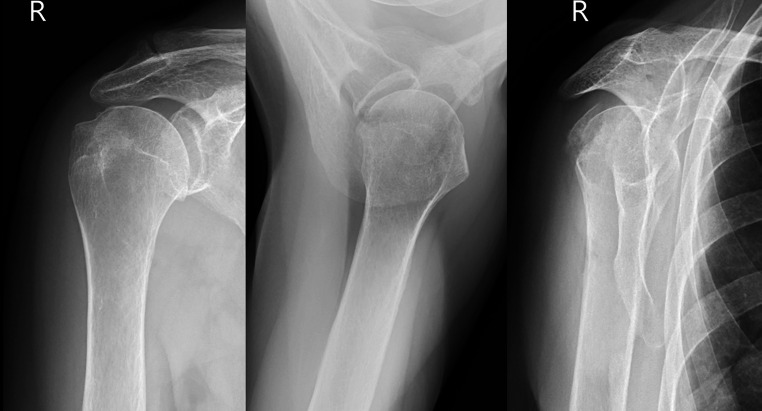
Basic trauma series of radiographs for evaluating a right proximal humerus fracture of 76 years old female with fracture of the greater tubercle adequately visible only on scapular Y view [37]
